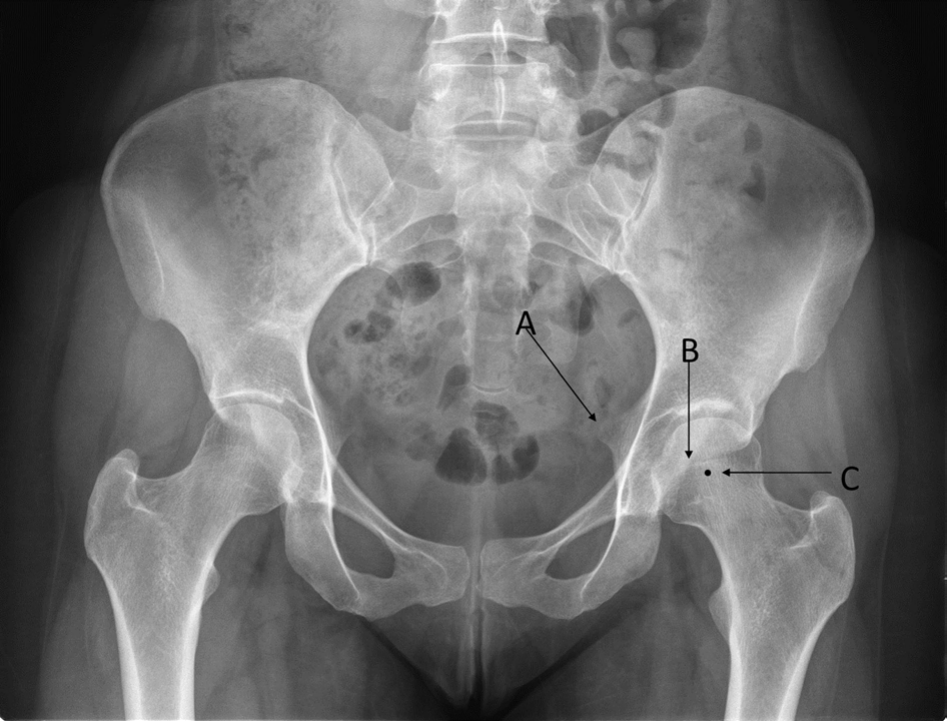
The radiographic findings of acetabular retroversion consist of a positive ischial spine sign (A), crossover sign (B), and a posterior wall sign (C). The small acetabular size and volume represents a challenge for surgical treatment. Adapted with permission from Dr. Rafael J. Sierra and the Mayo Foundation, Rochester, MN, USA.
